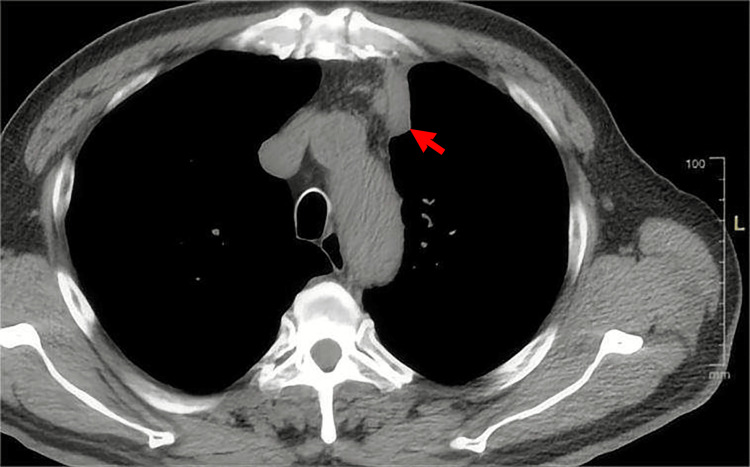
The patient’s follow-up chest CT scan revealed a notable decrease in soft tissue density in the left upper apical lobe compared to the initial admission scan, as indicated by the red arrow.
